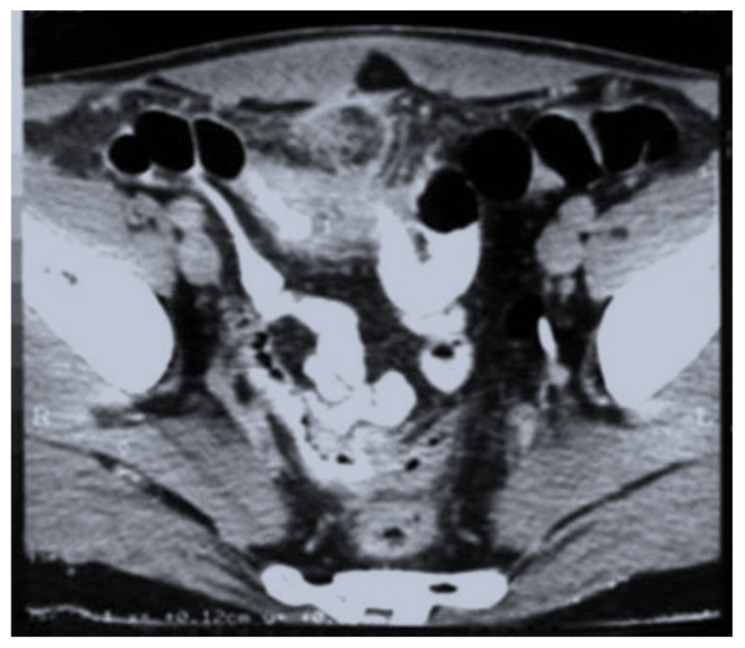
CT scan—heterogeneous mass involving distal ileum; inflammatory changes of the mesentery were also observed.
